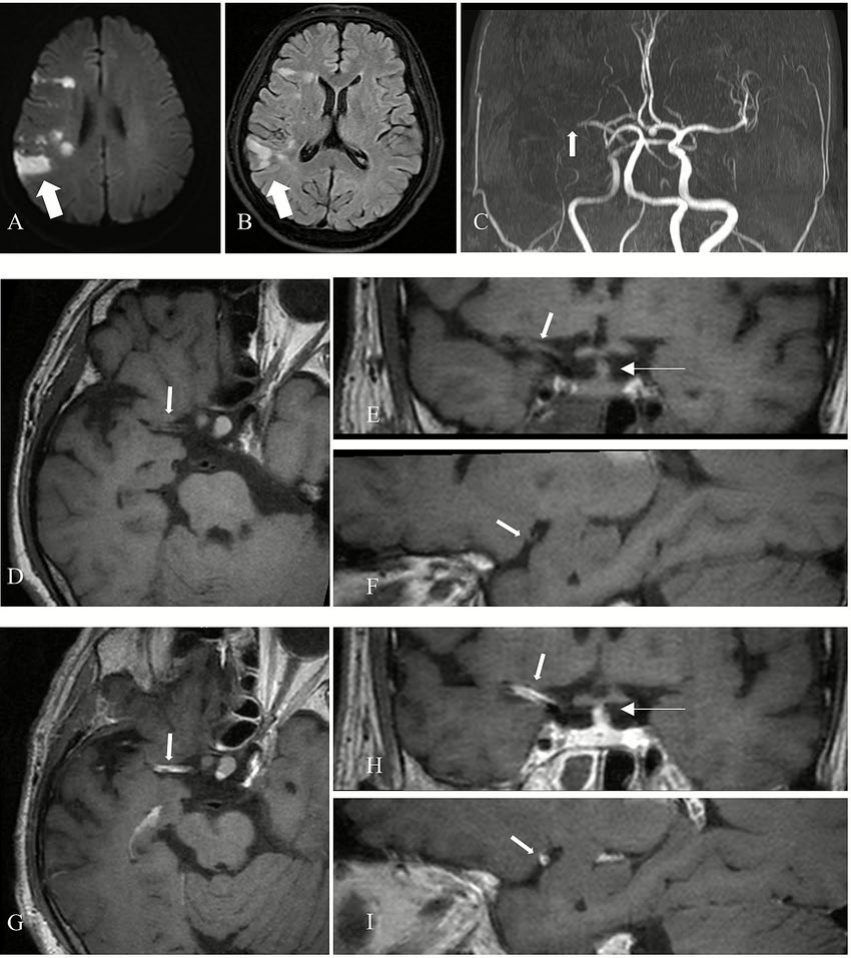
Grade II enhancement of a culprit plaque in a patient with acute cerebral infarction. (A) DWI shows acute cerebral infarction in the right frontal and parietal lobes (arrow). (B) FLAIR shows hyperintensity in the corresponding region (arrow). (C) MRA demonstrates severe stenosis in the right MCA (M1 segment) (arrow). (D,G) Axial pre- and post-enhancement contrast images identify the plaque in the right MCA (M1 segment) (arrow). (E) Coronal image shows the plaque (thick arrow) and the pituitary stalk (thin arrow). (H) Coronal post-contrast image shows the plaque (thick arrow) with a higher degree of enhancement than the pituitary stalk (thin arrow). (F,I) Sagittal reconstructions perpendicular to blood flow before and after contrast show the eccentric plaque (arrow).
